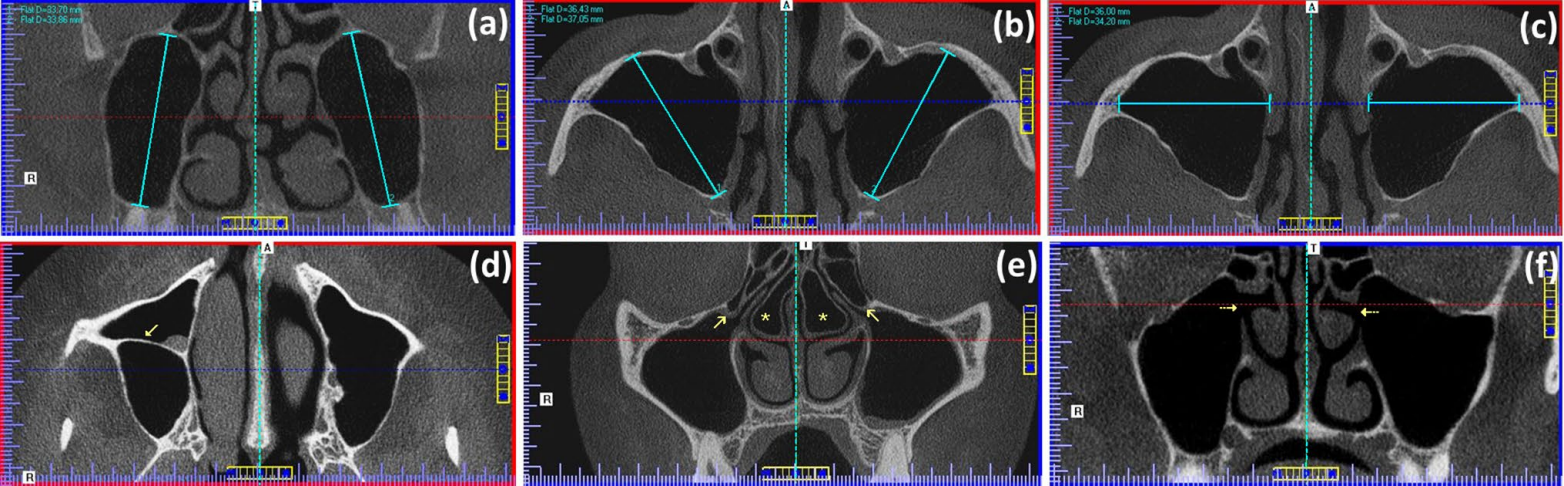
CBCT scans of MS and related anatomical structures. Pictures a–c show the way of the measurement of different dimensions after enhanced using the AxioVision^®^ software as follows: a maximal craniocaudal (height), b maximal anteroposterior (depth), and c maximal transversal (width) dimensions. Picture d represents an axial view demonstrating the presence of an antral septum (solid arrow). Image e depicts the anatomical location of the Haller cells (solid arrows) and concha bullosa (asterisks) as detected through coronal sections. Figure f corresponds to a coronal view demonstrating the presence of bilateral accessory maxillary ostia (dotted arrows)
